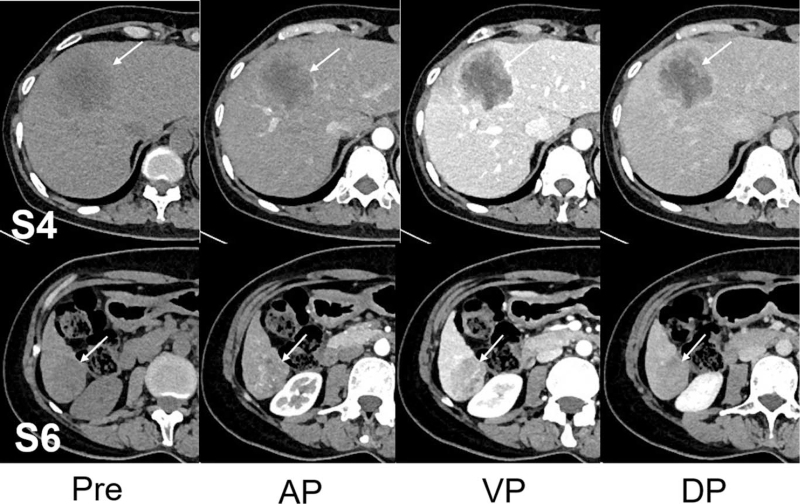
Abdominal computed tomography (CT) imaging of the 2 tumors (white arrows). Pre-contrast phase revealed 2 separate hypoattenuating hepatic masses in S4 and S6 (white arrows; 5.0 × 4.6 cm and 2.5 × 2.1 cm in size, respectively). On contrast-enhanced dynamic CT, the S4 tumor showed peripheral enhancement with central low density in the arterial phase and the peak enhancement appeared in the portal venous phase, and presented slightly centripetal enhancement in the delayed phase. The whole S6 tumor was heterogeneously enhanced in the arterial phase and followed by wash-out in the portal venous phase, and the delayed phase. AP = arterial phase, DP =  delayed phase, Pre = pre-contrast, VP = portal venous phase.
